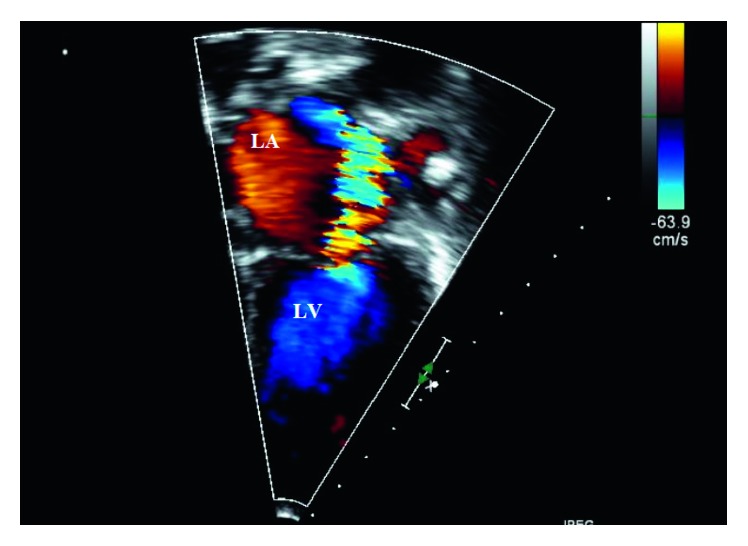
Transthoracic echocardiogram in apical four-chamber view demonstrating enlargement of the left atrium (LA) and severe mitral valve regurgitation.
